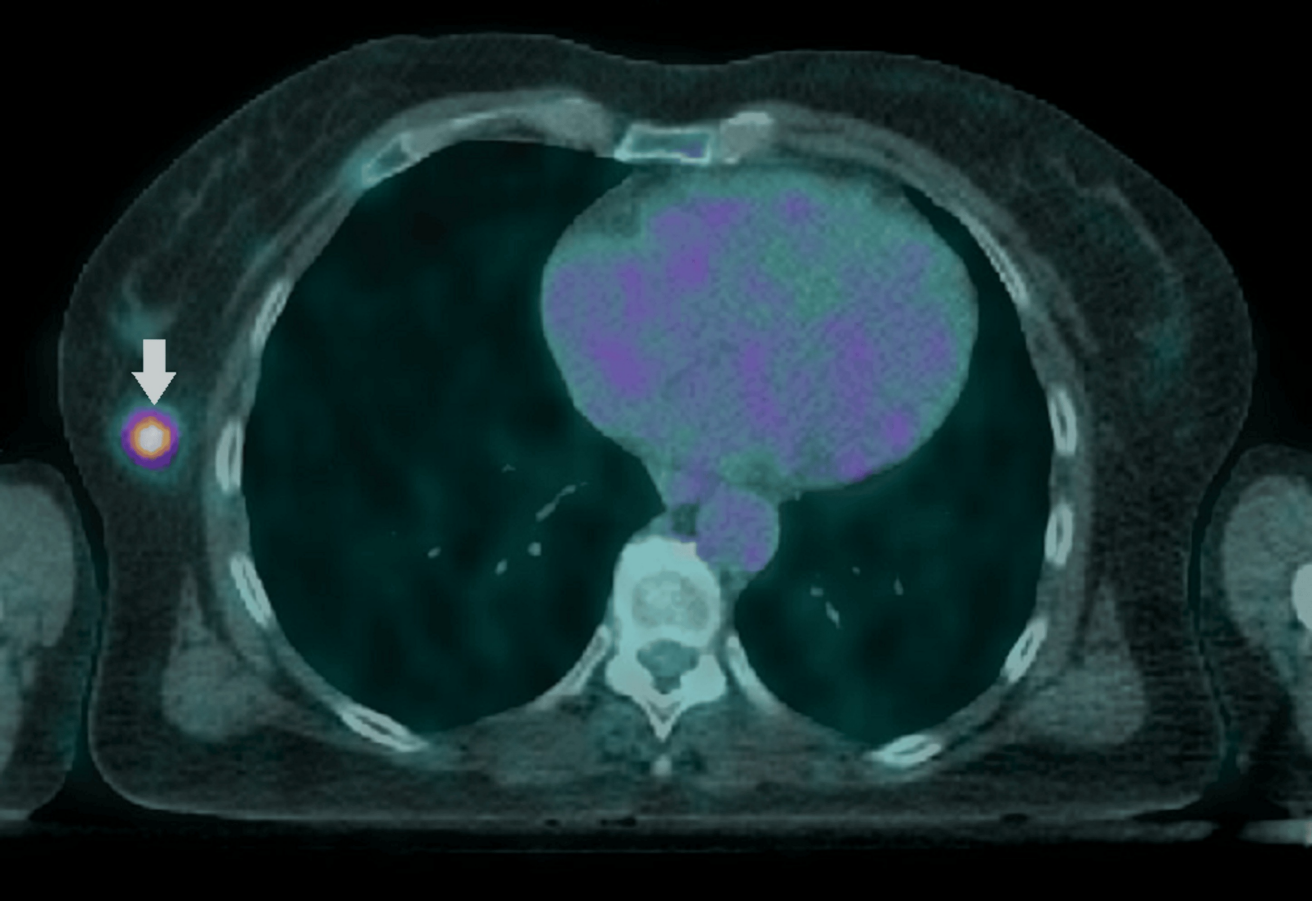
Staging 18F-FDG PET-CT showing hypermetabolic activity at the transition of the outer quadrant of the right breast 18F-FDG PET-CT: 18F-fluorodeoxyglucose positron emission tomography-computed tomography
